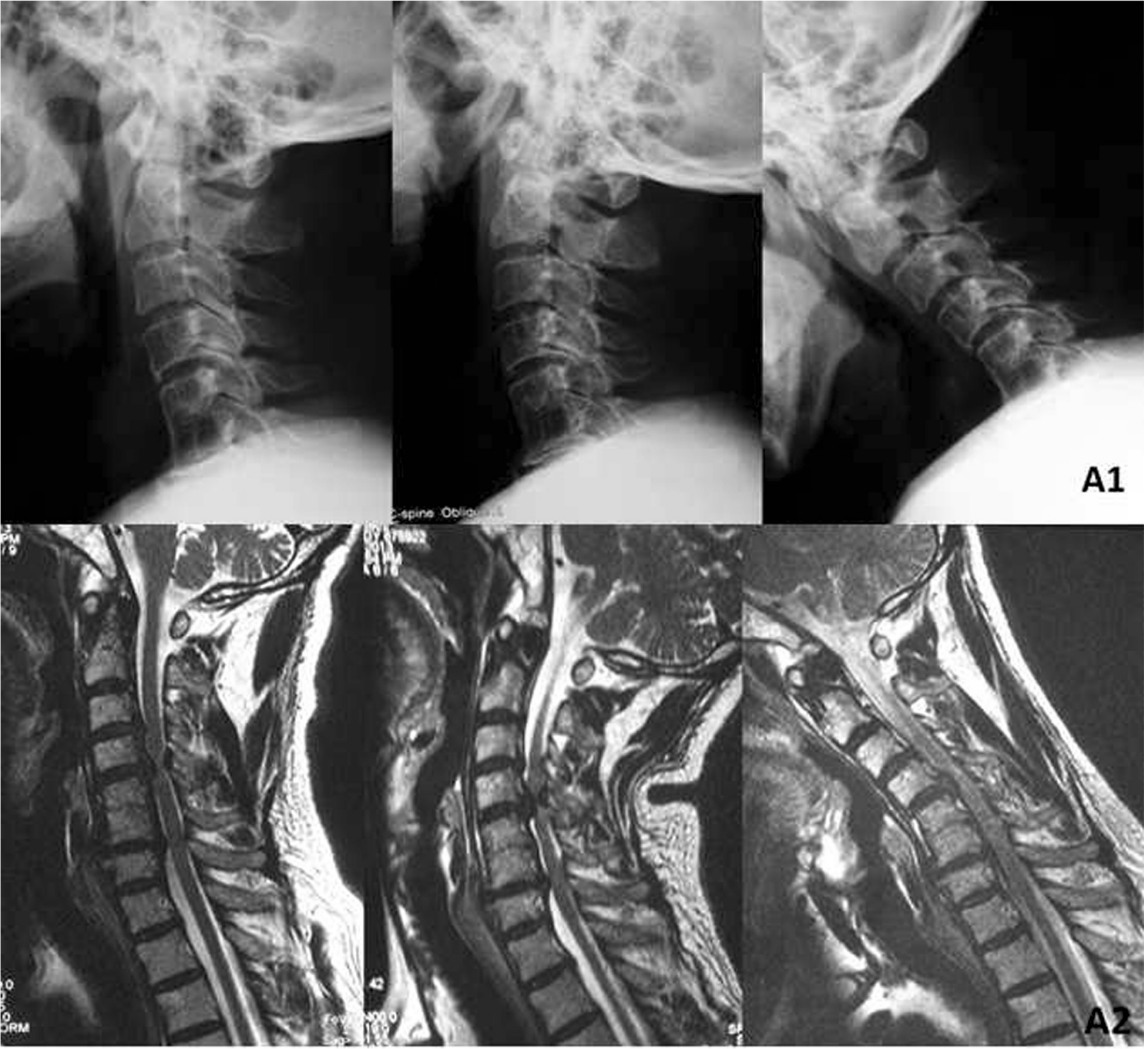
A 67-year-old man developed one-level adjacent segment disease 11 years after primary surgery. (*A1*) Radiograph and (*A2*) MRI (flexion/extension) at 11 years after operation indicates C3-4, C4-5 disc hernias
